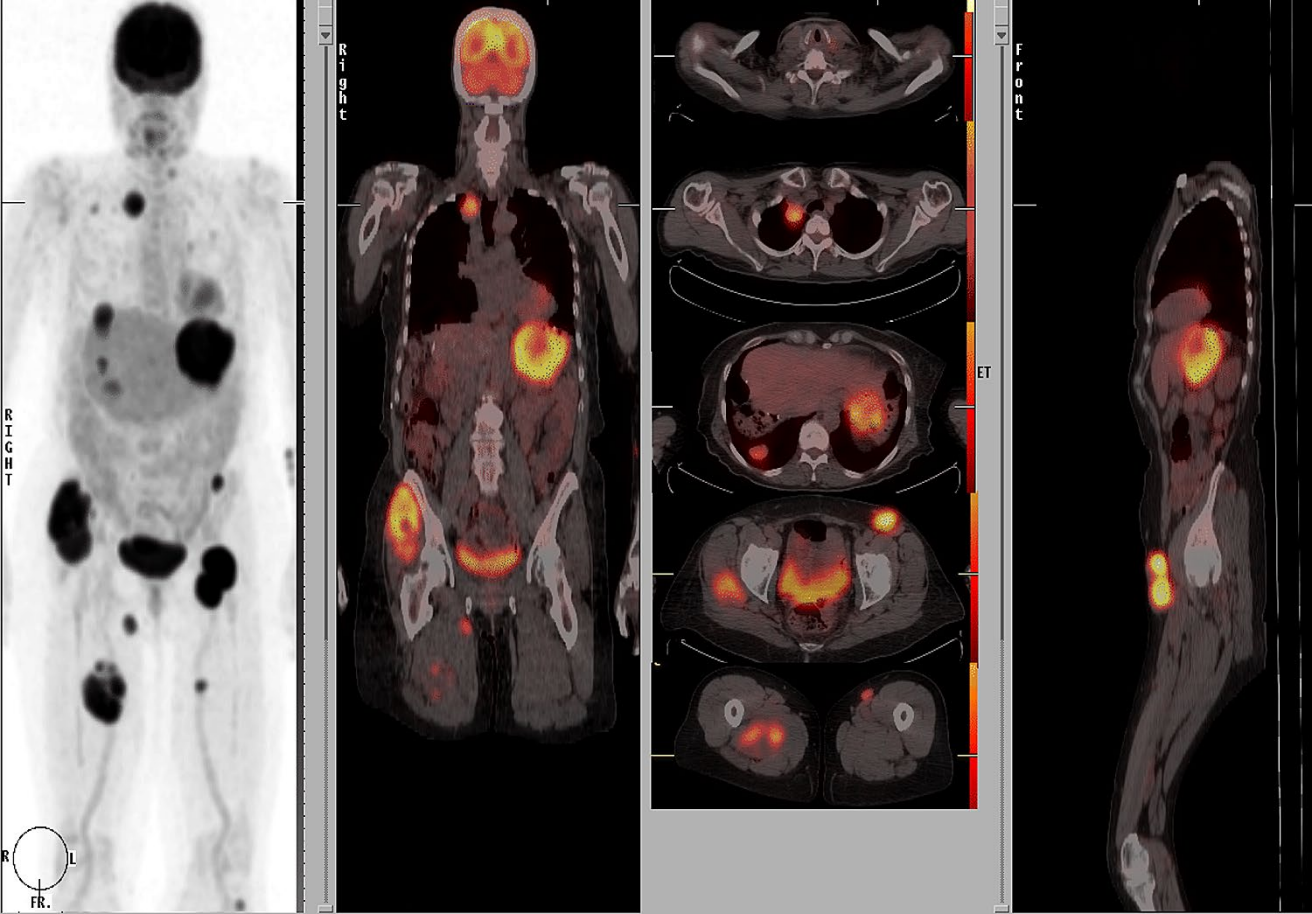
61 year old Caucasian woman with disseminated melanoma by PET/CT scan, previously published as a book cover, From Local Invasion to Metastatic Cancer: Involvement of Distant Sites Through the Lymphovascular System, Ed. Stanley P. L. Leong, Humana Press, 2009, New York, NY, USA
